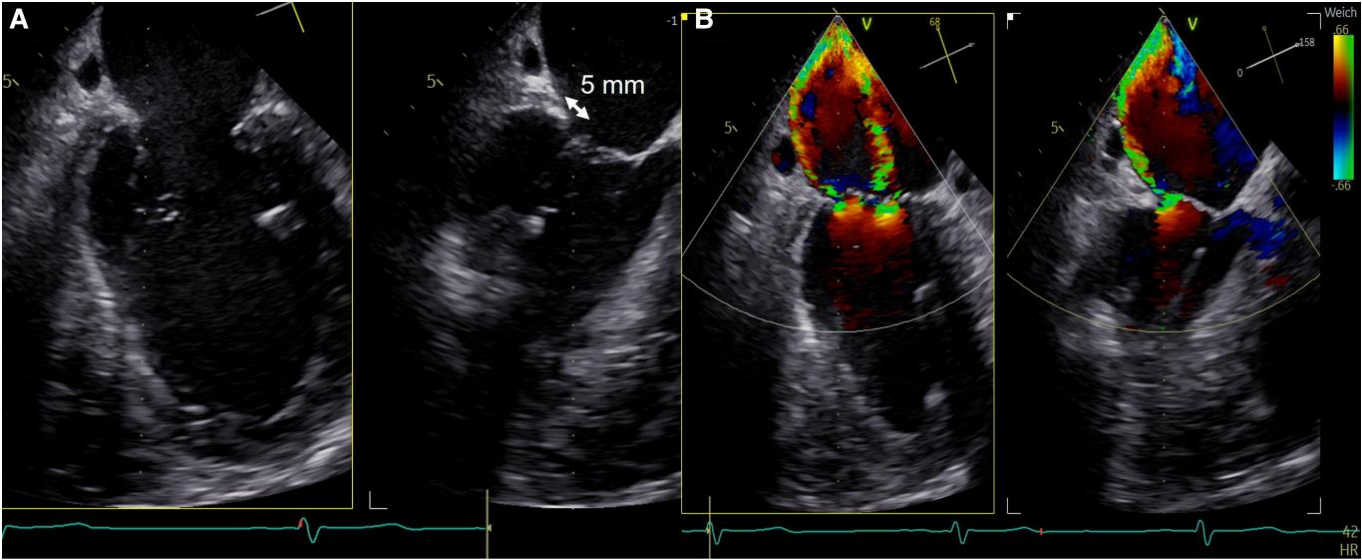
Baseline transoesophageal echocardiography, midesophageal commissural view of mitral valve. (*A*) Without colour Doppler, length of the posterior leaflet 5 mm; (*B*) severe eccentric mitral regurgitation. Before TMVI, there were no regional wall motion abnormalities of the LV with a left ventricular end-diastolic volume index of 84 mL/m².
